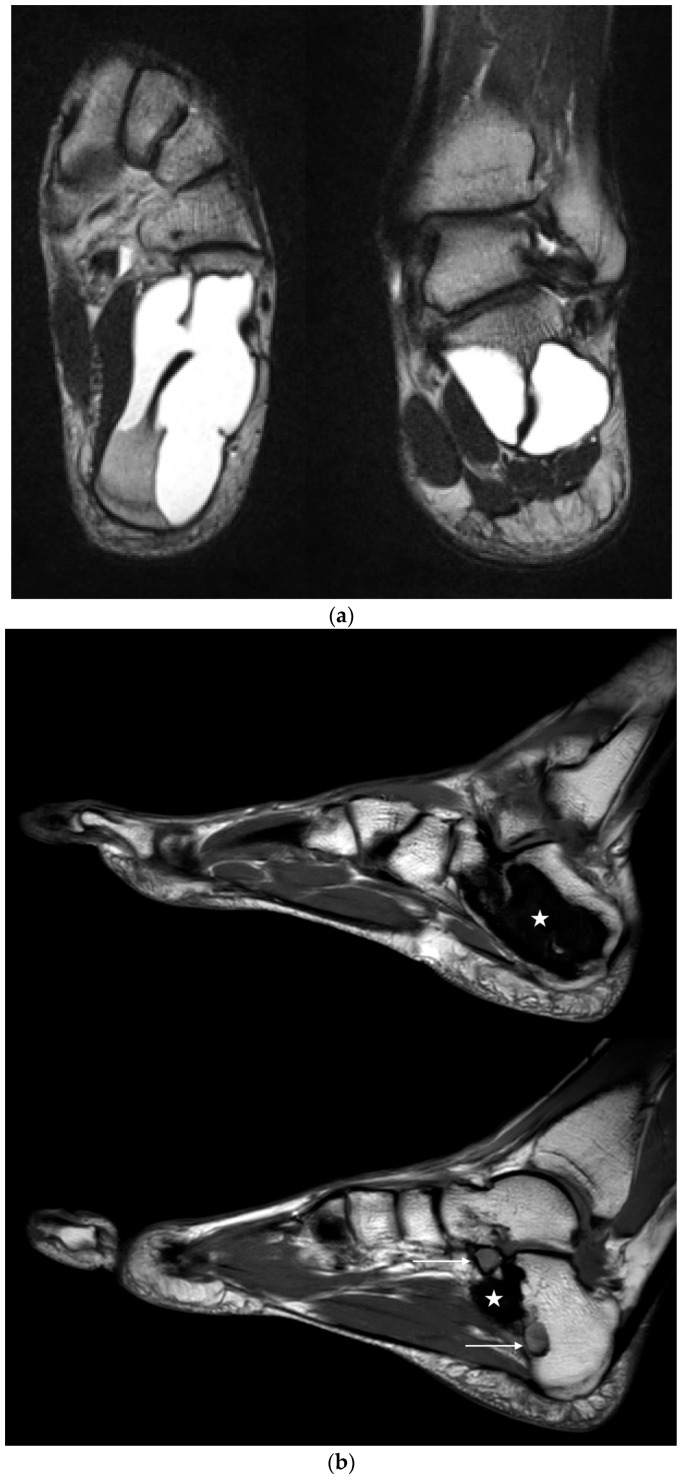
(a) Axial and coronal T2 TSE-weighted MRI showing a large SBC in a 31-year-old professional ballet dancer (case 6). After endoscopic resection, the bone cavity was filled with injectable bone substitute. (b) Sagittal T1-weighted MRI performed 91 months postop, showing two small areas of recurrence of SBC at the medial aspect (bottom image, arrows). The large cyst cavity was filled with QuickSet© injectable bone substitute (marked with a star), showing no signs of osseous remodeling more than 7.5 years after implantation. The patient is currently free of symptoms and still performs at the highest level of professional ballet dancing.
